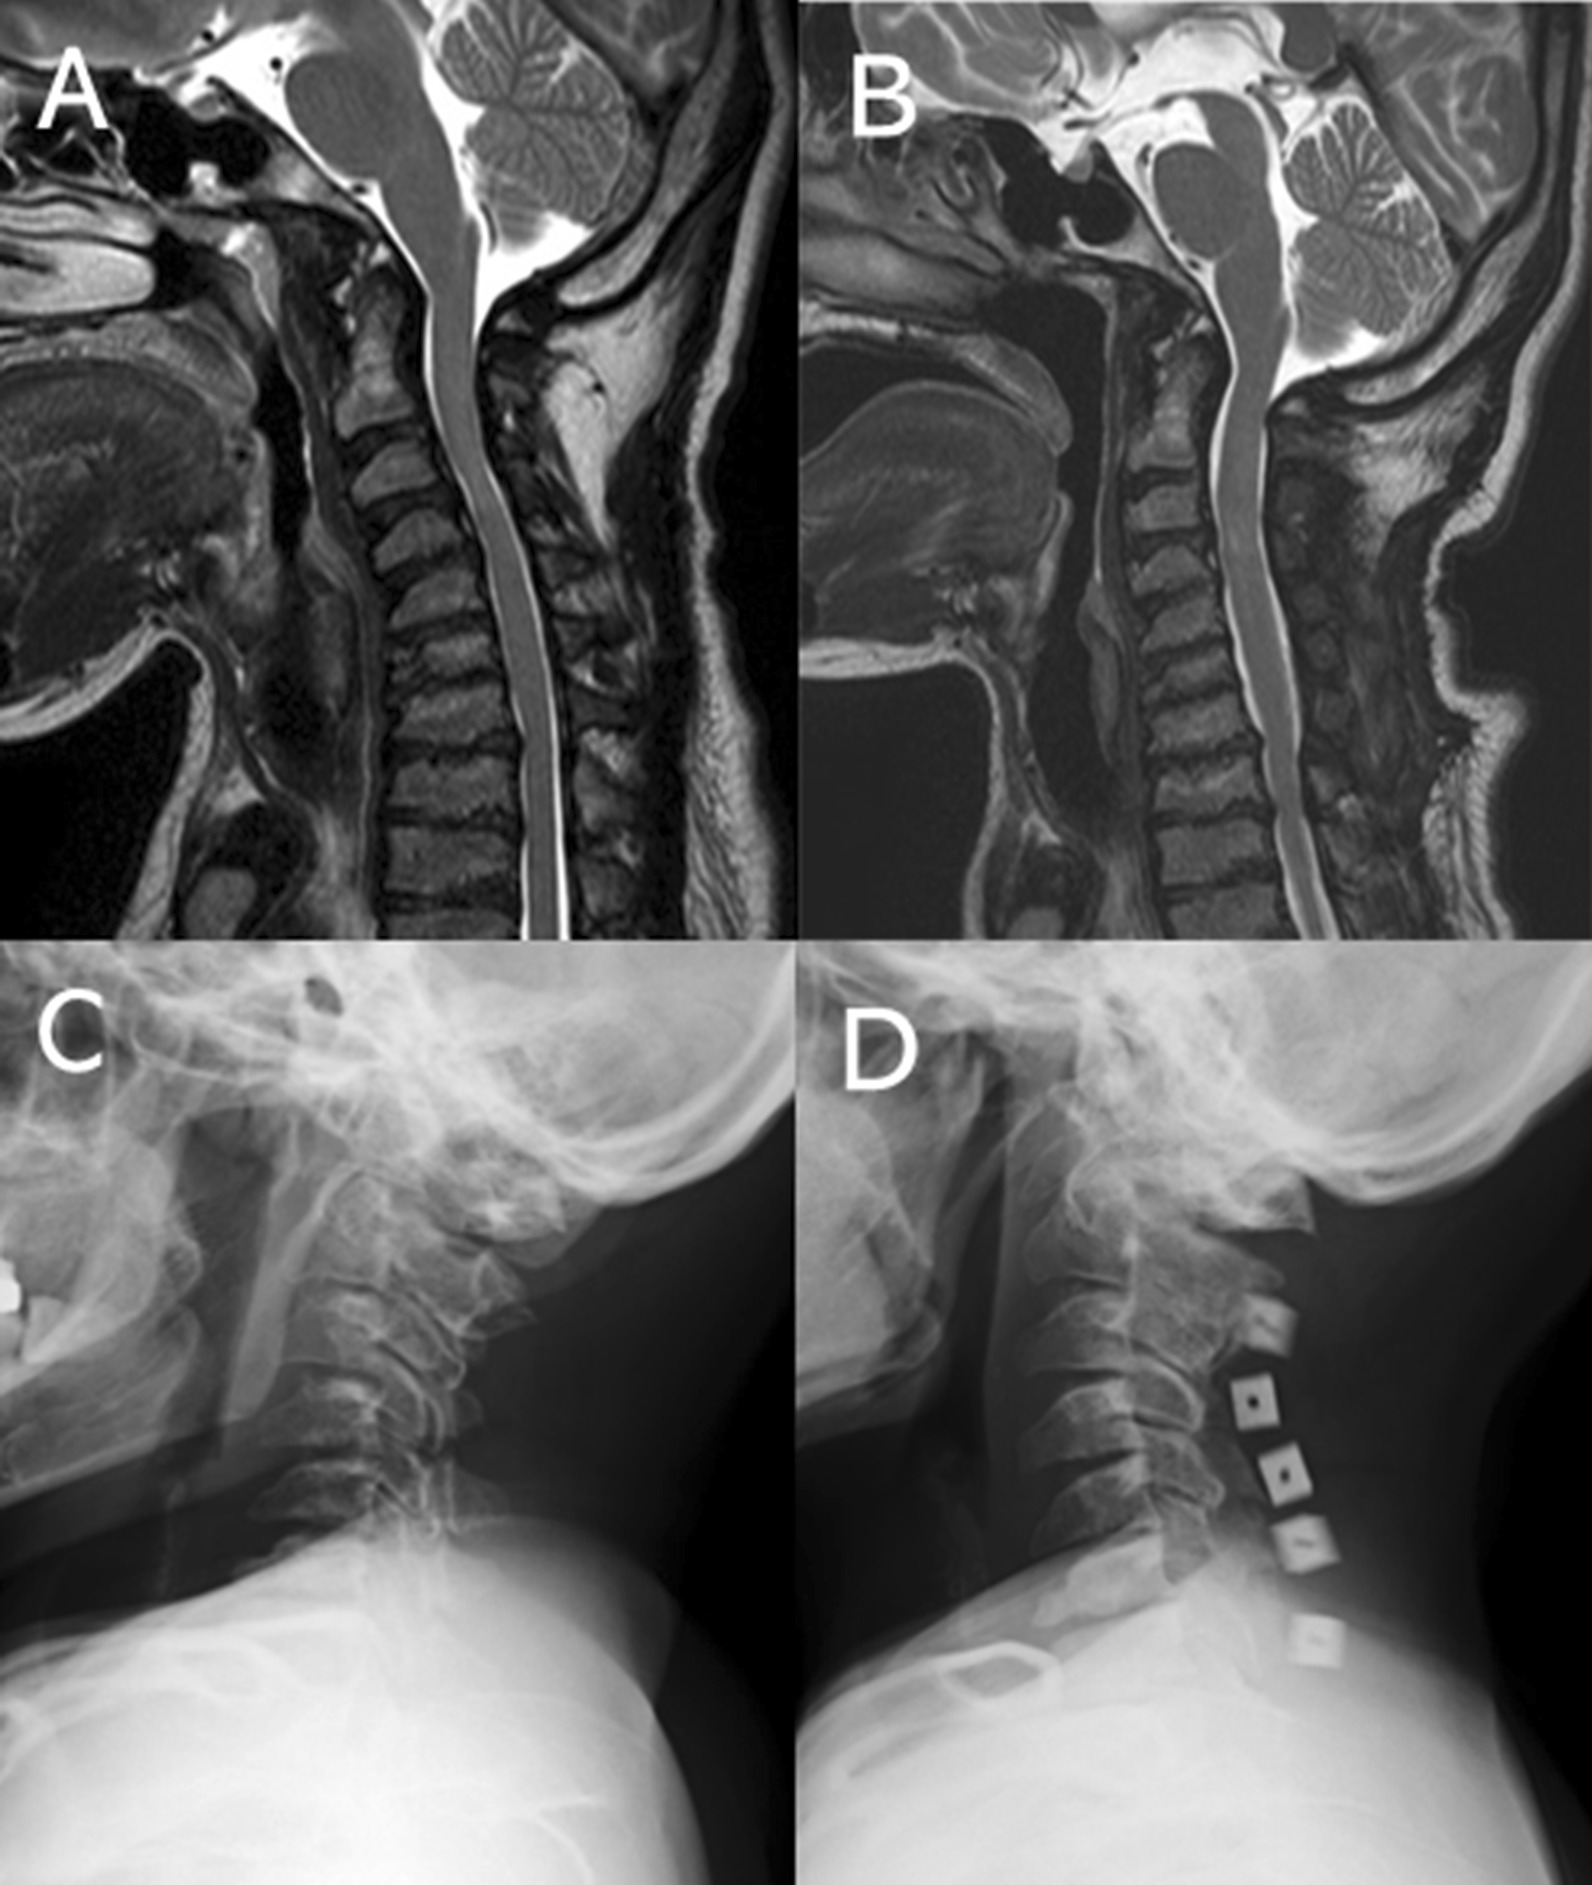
T2WI sagittal images and plain lateral radiographs of a 38-year-old female patient with ML type III. Her symptoms include clumsiness and gait disturbance. A, C Before operation. B, D Two years postoperatively. The sagittal cervical alignment became lordotic after surgery, which was maintained until the final follow-up (6 years postoperatively). Her myelopathic symptom disappeared immediately after surgery. *ML* mucolipidoses
